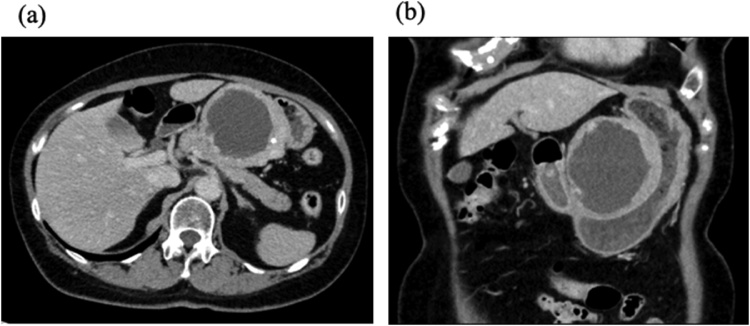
Abdominal enhanced computed tomography. a/b. A smooth-surfaced tumor measuring 70 × 70 mm is noted at the lesser curvature of the middle stomach. The tumor has an internal low-density area and a thick peripheral high intensity area which has an unclear boundary between the stomach. The tumor was suspected to be a submucosal tumor.
